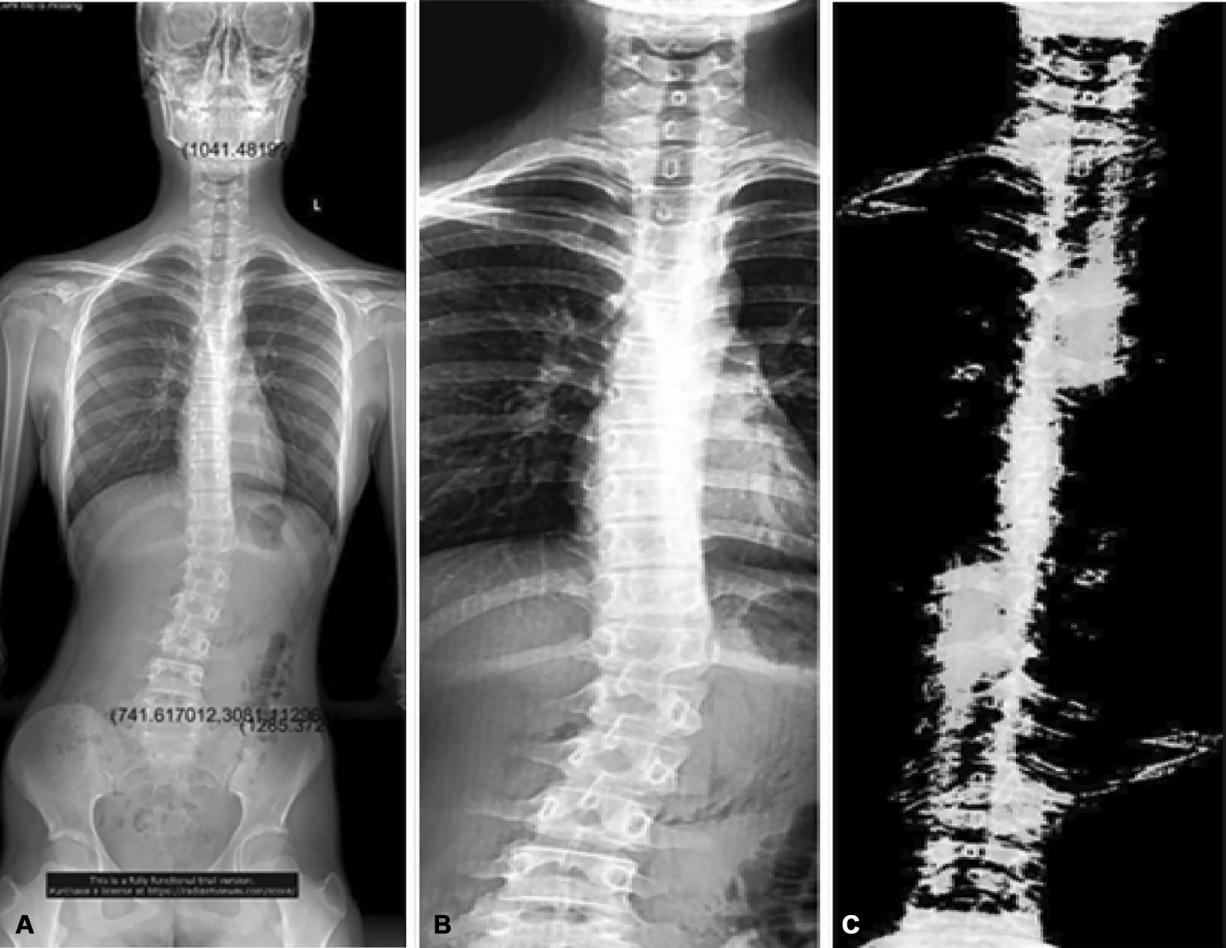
Spinal radiograph of the full spine, enhanced spine, and symmetrical superposition of the spinal region. (A) Full spine upstanding radiograph. (B) Enhancement image of the spinal region. (C) Spinal symmetric superposition imaging.
